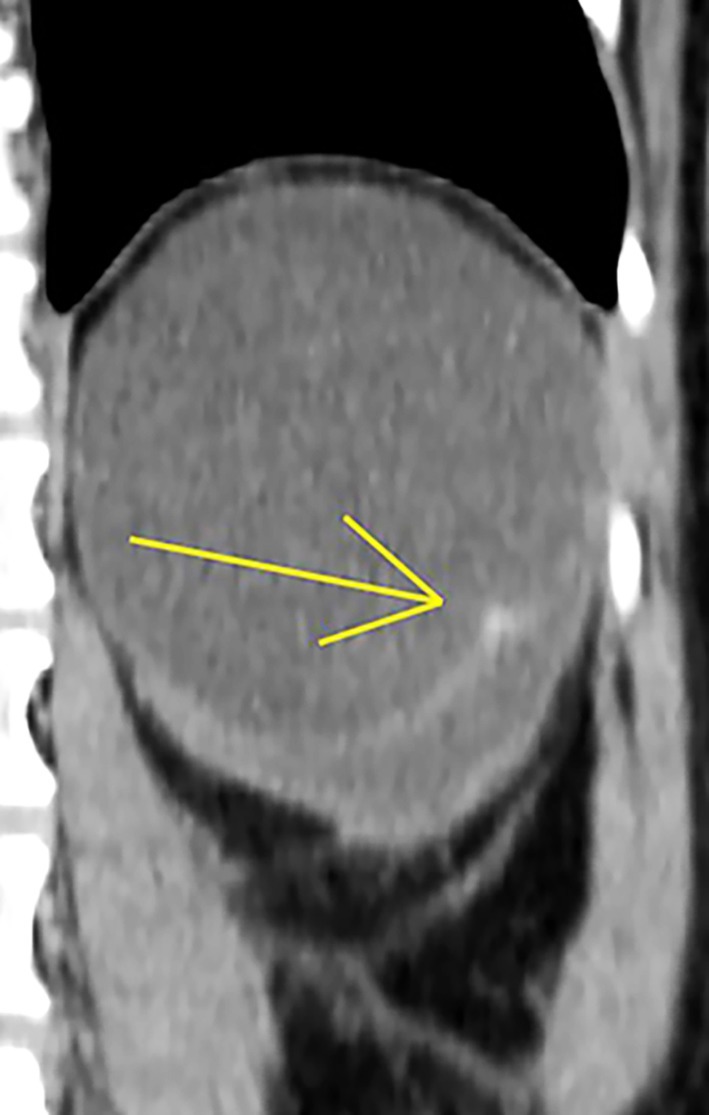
Computed tomography scan showing a cystic lesion with a contrast‐enhanced mural nodule within the left kidney.
